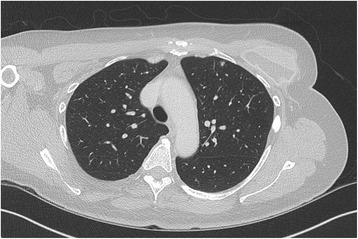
Computed tomography of the thorax demonstrating a small (5 mm in diameter) subpleural nodule within the anterior left upper lobe, which remained unchanged since the previous scan
